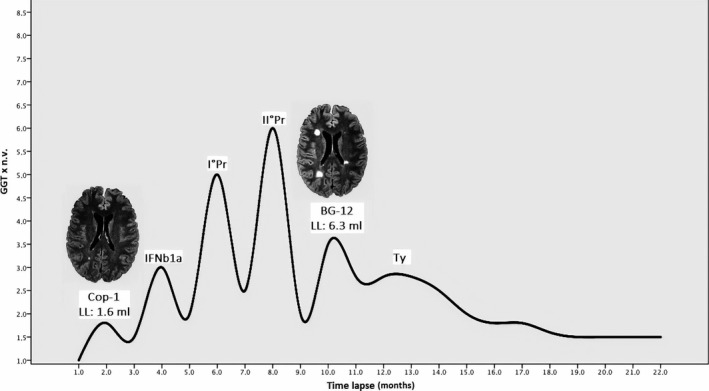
The graphic representation of the hepatic cytolytic indexes expressed as multiples of gamma‐glutamyl transferase (GGT) normal value (n.v.) during the first pregnancy (IPr), the second pregnancy (IIPr), and the drug administration: glatiramer acetate, interferon (IFNb1a), dimethylfumarate, and natalizumab (Ty). Note the magnetic resonance imaging of the brain with its lesion load (LL) expressed in milliliters (mL) at the start therapy and the last therapy before the natalizumab
